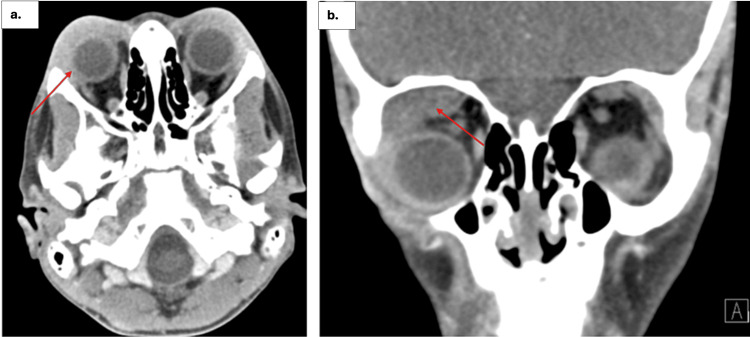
A CT scan of the orbit/sella/ear (with contrast) taken 18 months prior a. The axial CT scan revealed asymmetric proptosis, with greater enlargement of the lacrimal gland on the right side compared to the left. The enlarged right lacrimal gland is indicated by a red arrow. b. The coronal CT scan also demonstrated the enlargement of the right lacrimal gland. In this view, the enlarged gland is similarly pointed out with a red arrow. In both the axial and coronal planes, the red arrows highlight the enlargement of the right lacrimal gland, with no evidence of an abscess.
